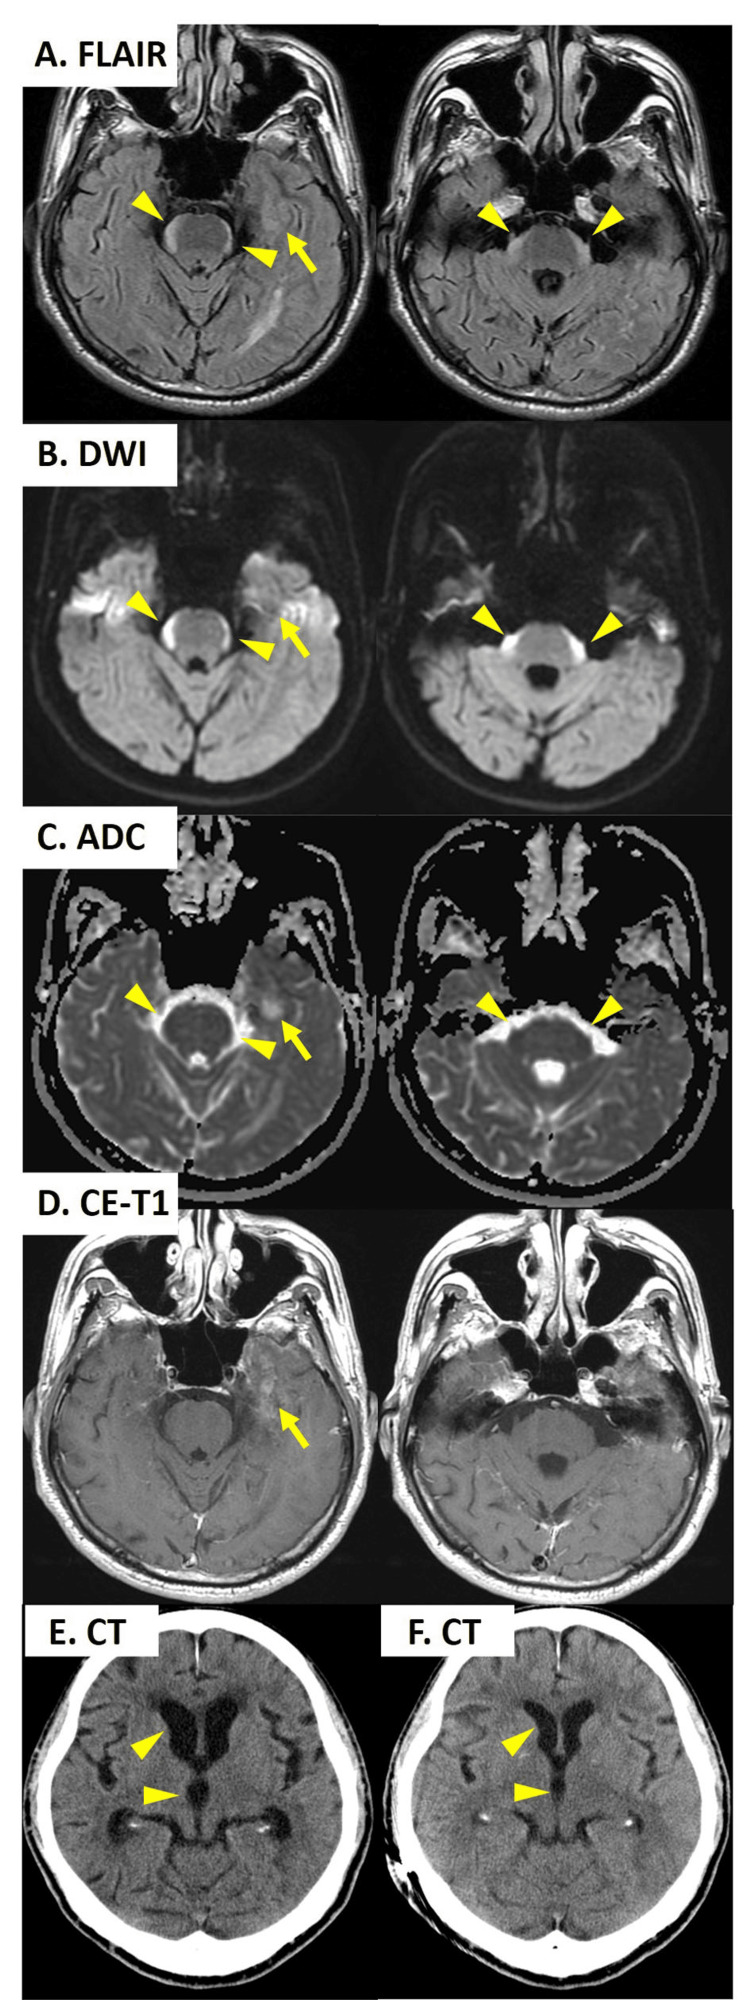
Brain magnetic resonance imaging (MRI) findings. (A) Fluid attenuation inversion recovery (FLAIR) imaging shows a band-like high-intensity lesion surrounding the ventral brainstem (arrowheads) and a slight high-intensity lesion in the left inner temporal lobe (arrow). (B) Diffusion-weighted imaging (DWI). (C) Apparent diffusion coefficient (ADC) mapping. The brainstem lesion shows a restricted pattern of the high intensity on DWI and low on ADC (arrowheads). Conversely, the left temporal lesion shows an unrestricted diffusion pattern (arrow). (D) Contrast-enhanced T1 weighted image (CE-T1). The brainstem lesion is not enhanced with the gadolinium contrast agent while the left inner temporal lesion is slightly enhanced (arrow). (E, F) Head computed tomography (CT) findings. (E) CT shows mild ventriculomegaly of the lateral and third ventricles before the ventriculoperitoneal shunt (arrowheads). (F) After the shunt, the ventriculomegaly improves (arrowheads).
